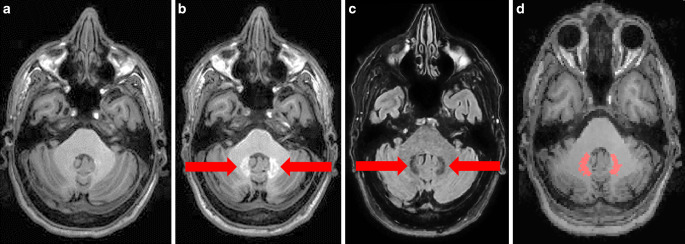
A patient with visible T1w signal intensity increase within the dentate nucleus is shown. a N_linear GBCA_ = 0, N_linear GBCA_ = 19, c FLAIR image, d dentate nucleus mask. Native T1w images were acquired in 2009 (a) and after 20 applications of linear gadolinium-based contrast agents (GBCA), in 2016 (b); the dentate nucleus appears brighter (*arrows*); from the FLAIR image (c) and the dentate nucleus mask of FSL SUIT toolbox (not shown), the customized dentate nucleus mask was generated and projected on the normalized T1w image (d, *mask in red*)
